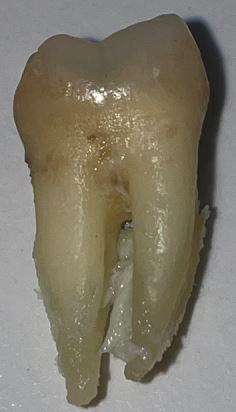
Full procedural stages including: Tooth and root assessment Access Canal identification Biomechanical preparation (De)obturation Post preparation Restoration	Fully anatomical including narrow canals/sclerosis Excellent tactile feedback from different dental tissues during access and preparation Real-world dentine colour changes for canal identification Low cost	Implications under the Human Tissues Act 2004 Limited standardisation across student cohorts Inability to control or predict potential challenges (eg pulp stones) Variable intra- and inter-year availability Radiographic interpretation required for identification of procedural errors and technical quality of obturations Cross-infection and disposal
